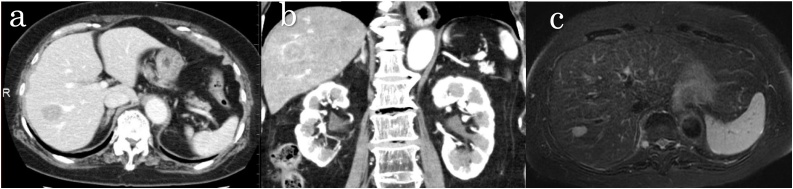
Axial (a) and coronal (b) contrast-enhanced CT images show a poorly enhancing mass in segment 7 of the liver. (c) Fat suppressed T2-weighted MR image shows slightly hyperintense mass in segment 7 of the liver.
